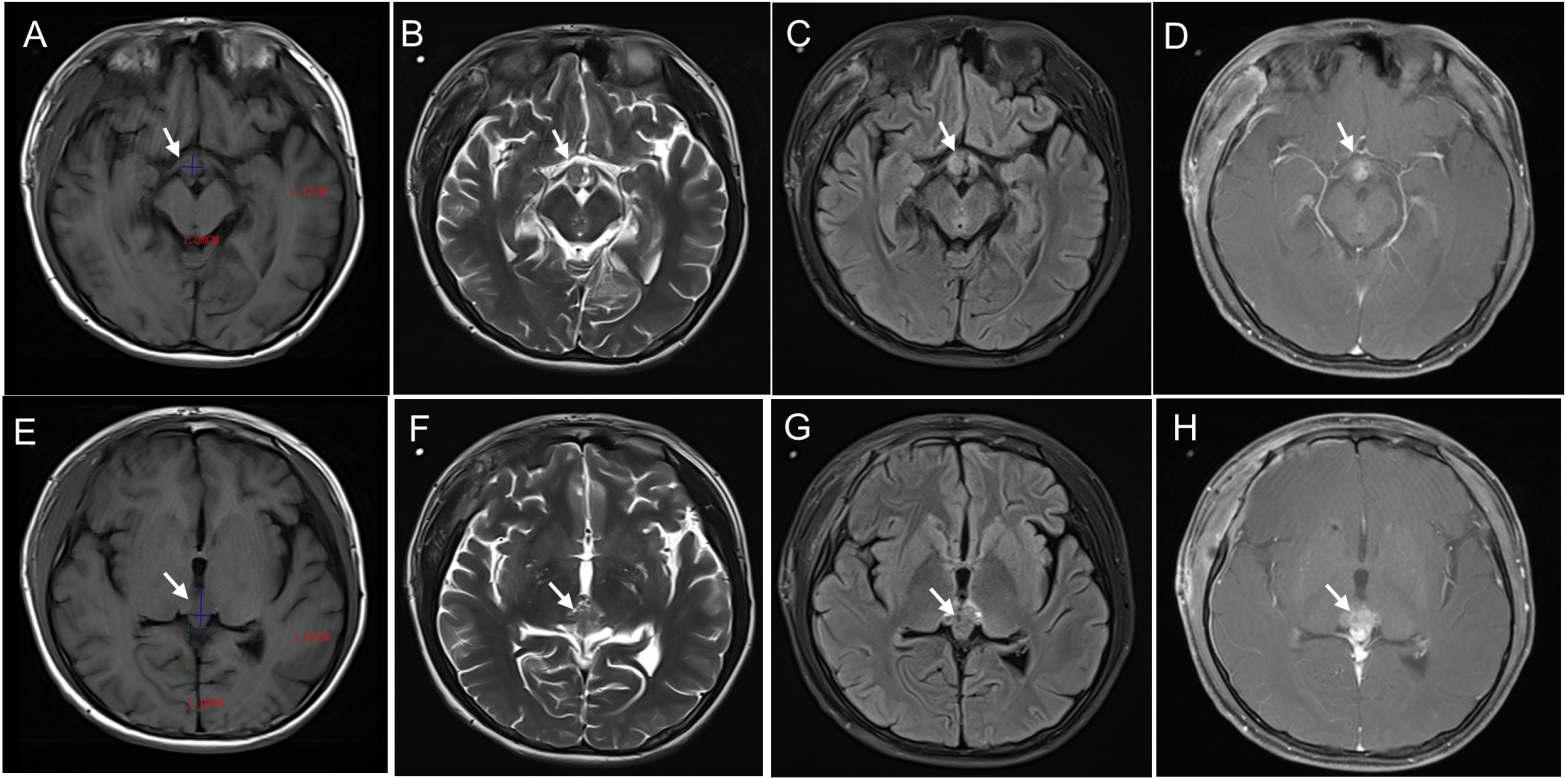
Cranial MRI manifestations of a mixed germ cell tumor in the suprasellar region (A-D) and pineal region (E-F) . On T1-weighted imaging sequences, the tumor displayed iso- to slightly hypointense signals (A, E). On T2-weighted imaging (B, F) and fluid-attenuated inversion recovery (FLAIR) sequences, it exhibited iso- to slightly hyperintense signals. Heterogeneous enhancement was observed on post-contrast images (D, H).
